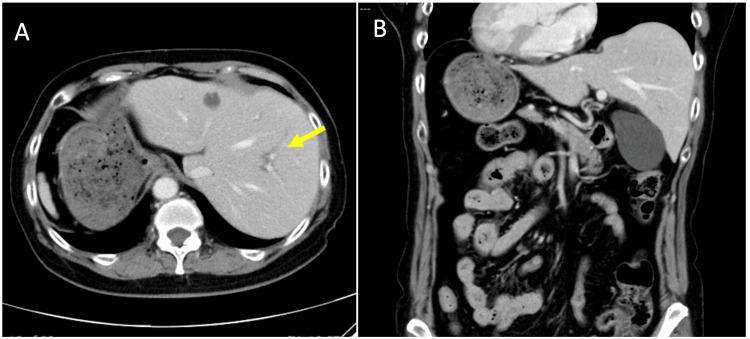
Contrast-enhanced abdominal computed tomography. A: Axial view showing a left-sided liver and right-sided stomach, consistent with mirror-image anatomy. Mild dilation of the intrahepatic bile ducts is also noted (yellow arrow). B: Coronal view confirming complete transposition of the thoracoabdominal organs, including dextrocardia.
